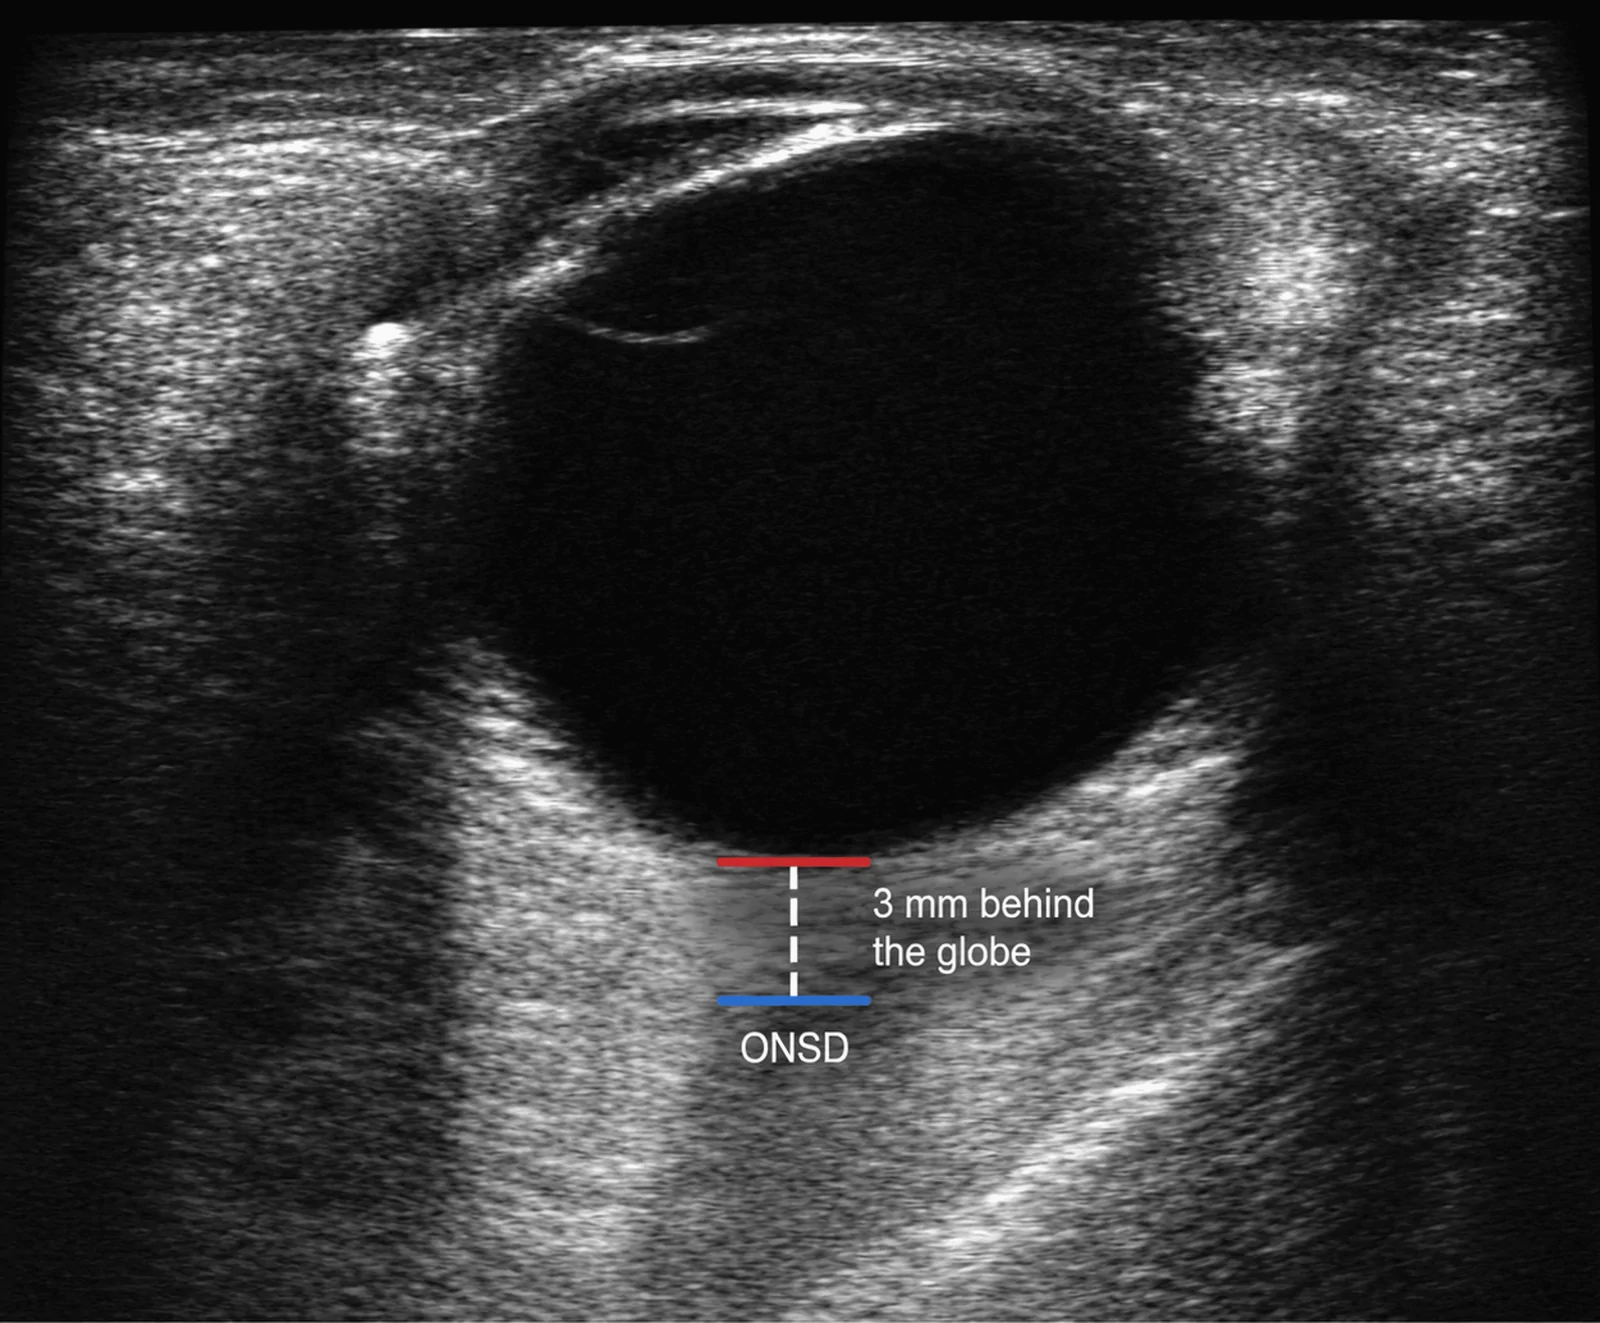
Optic nerve sheath diameter (ONSD) measured by a linear ultrasound probe Original image obtained using SonoSite M-Turbo (FUJIFILM Sonosite, Inc., Bothell, USA)
